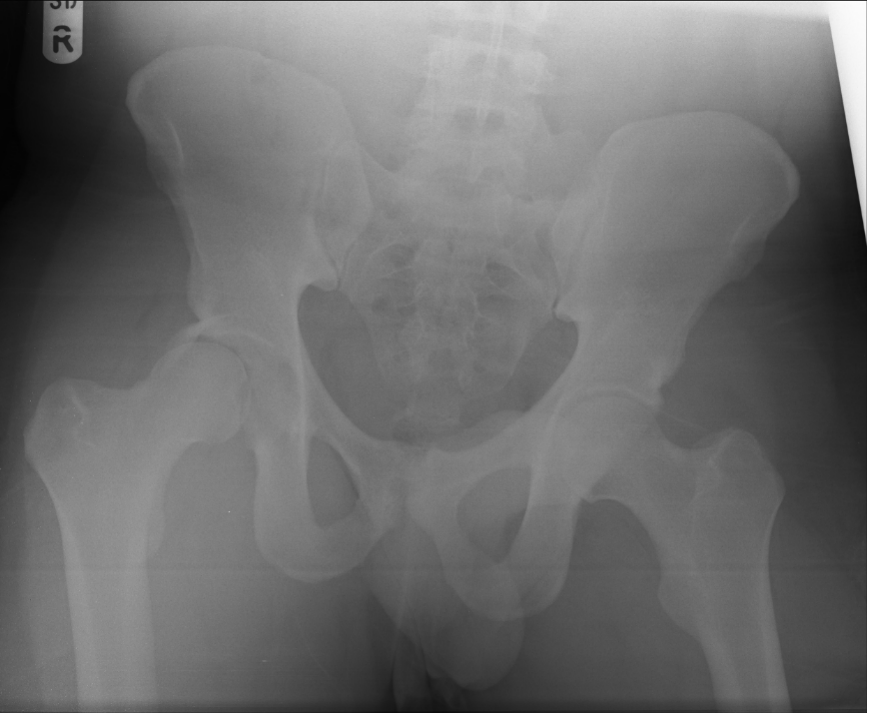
Anteroposterior pelvis radiographs.
